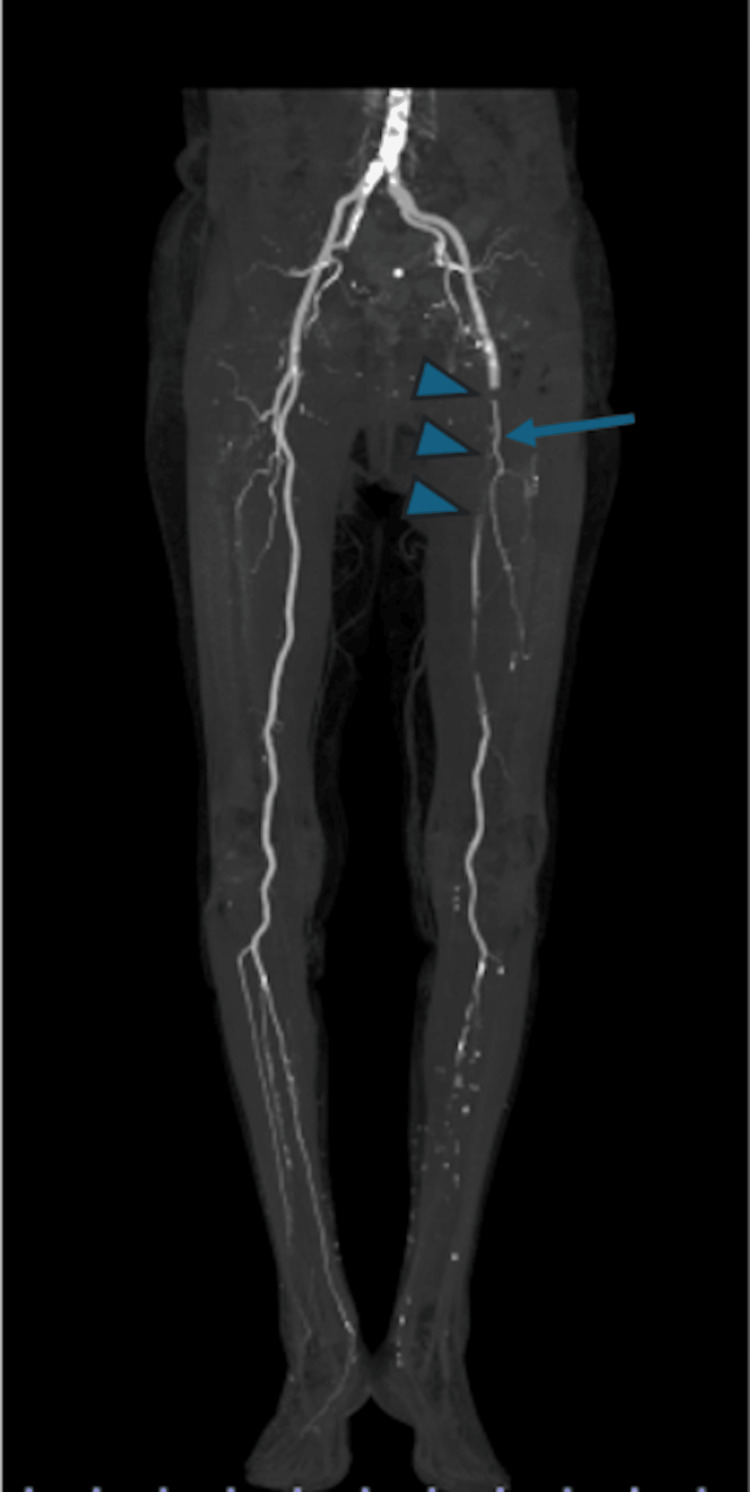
Preoperative contrast-enhanced CT angiography Contrast-enhanced computed tomography angiography shows obstruction of the left superficial femoral artery (SFA) from the ostium (arrow heads). Contrast enhancement was observed in the branches of the deep femoral artery (arrow). The collateral circulation of the SFA was poor.
